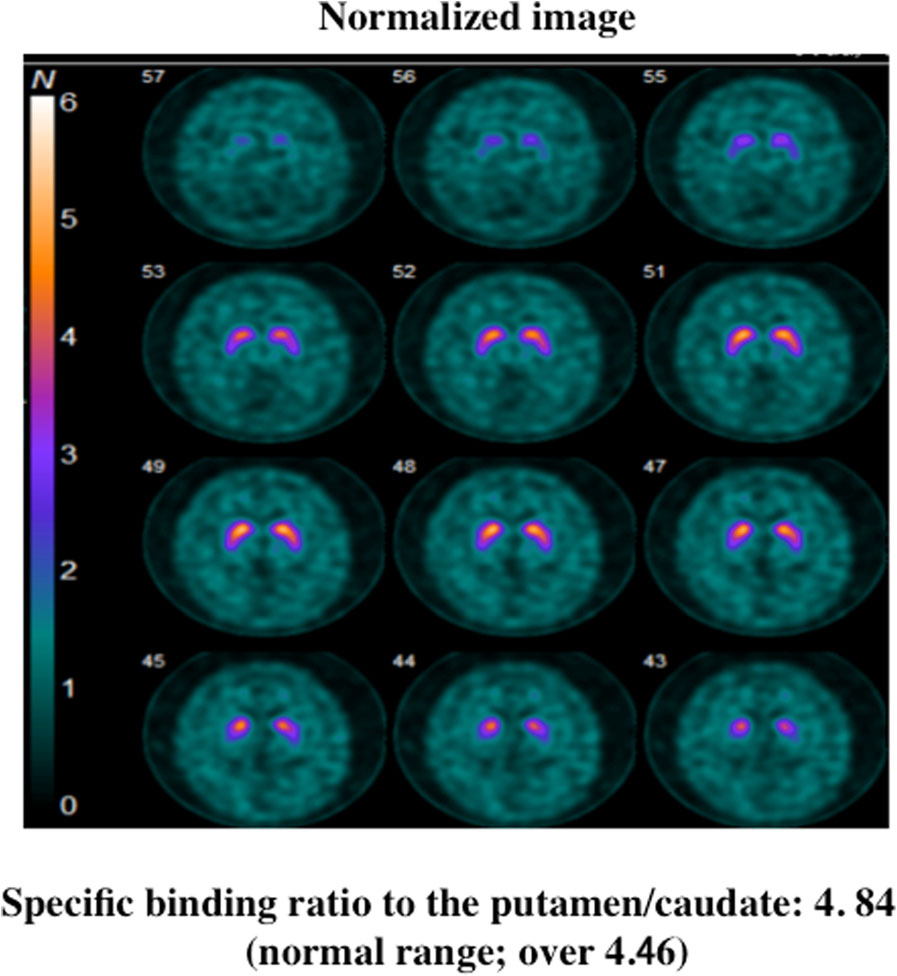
Dopamine transporter scintigraphy with ioflupane in this case. The significant reduced uptake was not confirmed in putamen or caudate nucleus
